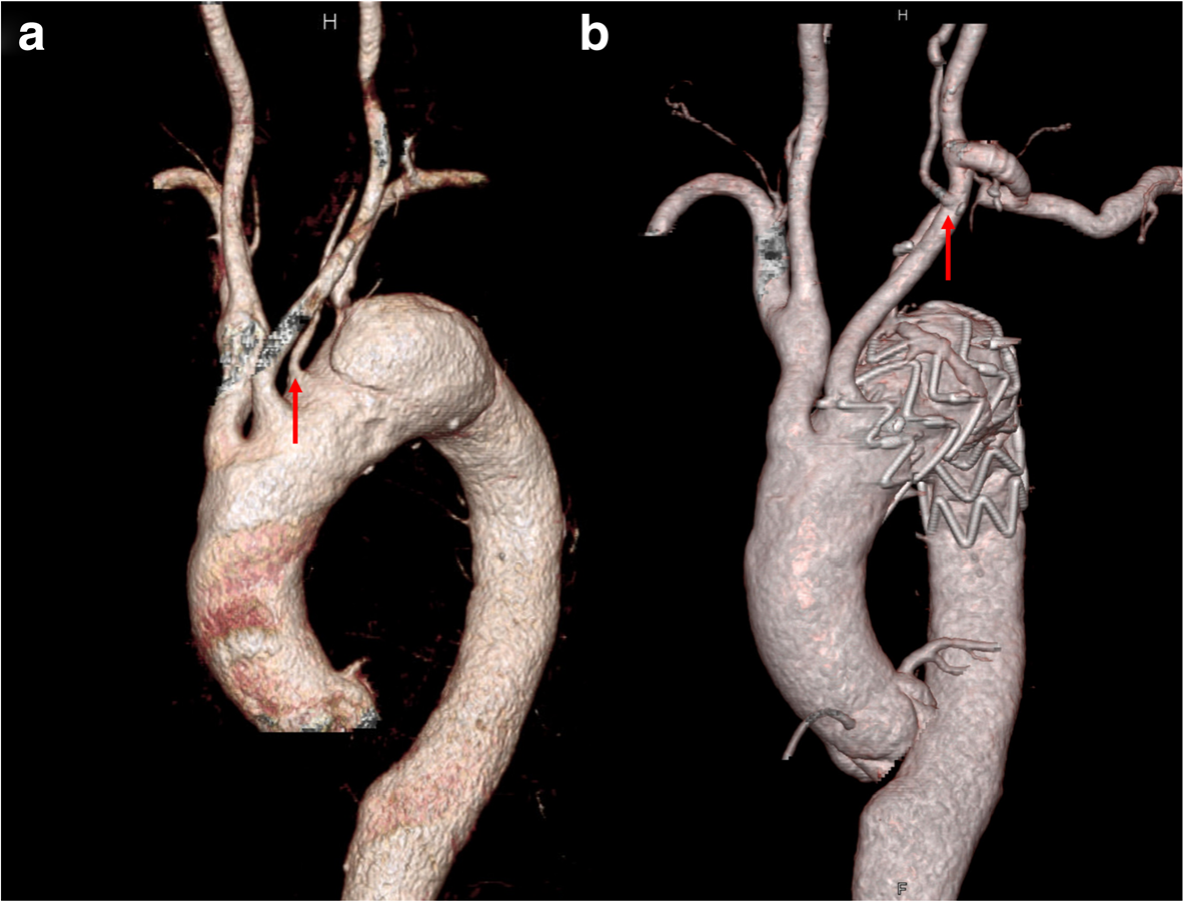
Chest computed tomographic (CT) angiography. a Preoperative CT angiography, the aberrant left vertebral artery (solid arrow) from aortic arch b Postoperative CT angiography, the transposed left vertebral artery (soild arrow) to the left common carotid artery
